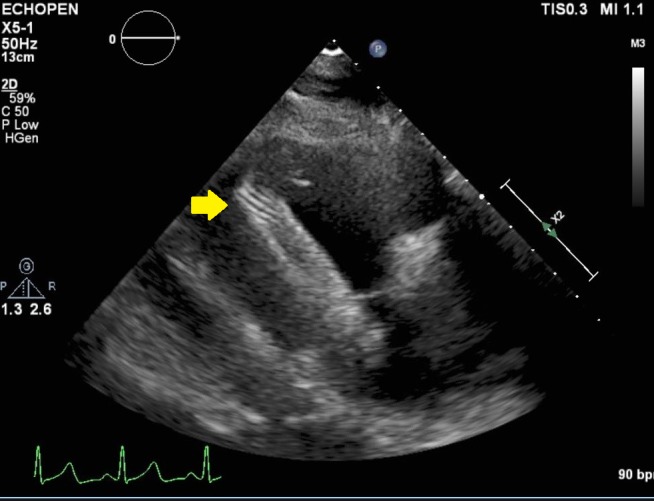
Right ventricular inflow view on trans-thoracic echocardiogram showing intracardiac stent in right ventricle.
